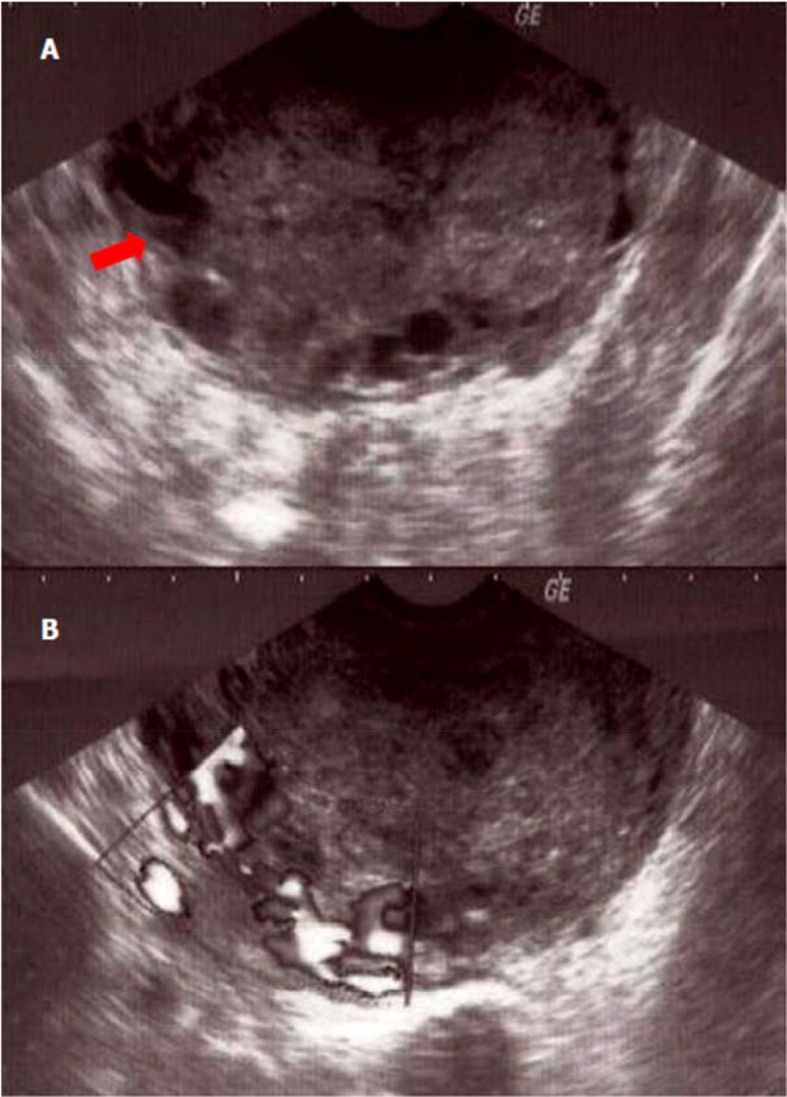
Sonographic appearance of the tumor of the placental site tumor. (A) Echogenic tumor occupying the uterine cavity (arrow) with infiltration of the myometrium; (B) appearance vascularized tumor at color Doppler.
